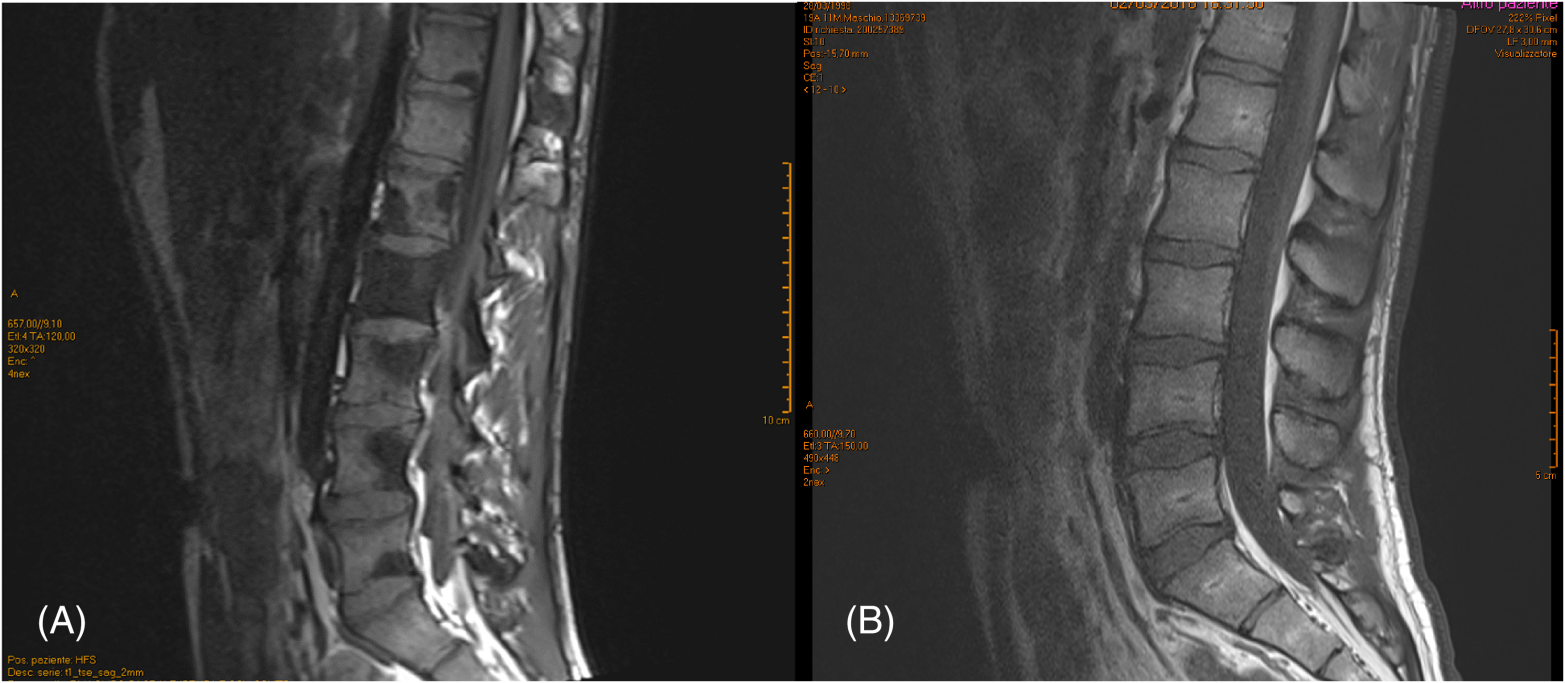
Spinal MRI showing vertebral bone metastase at diagnosis (A) and after 30 months of treatment with Crizotinib (B)
